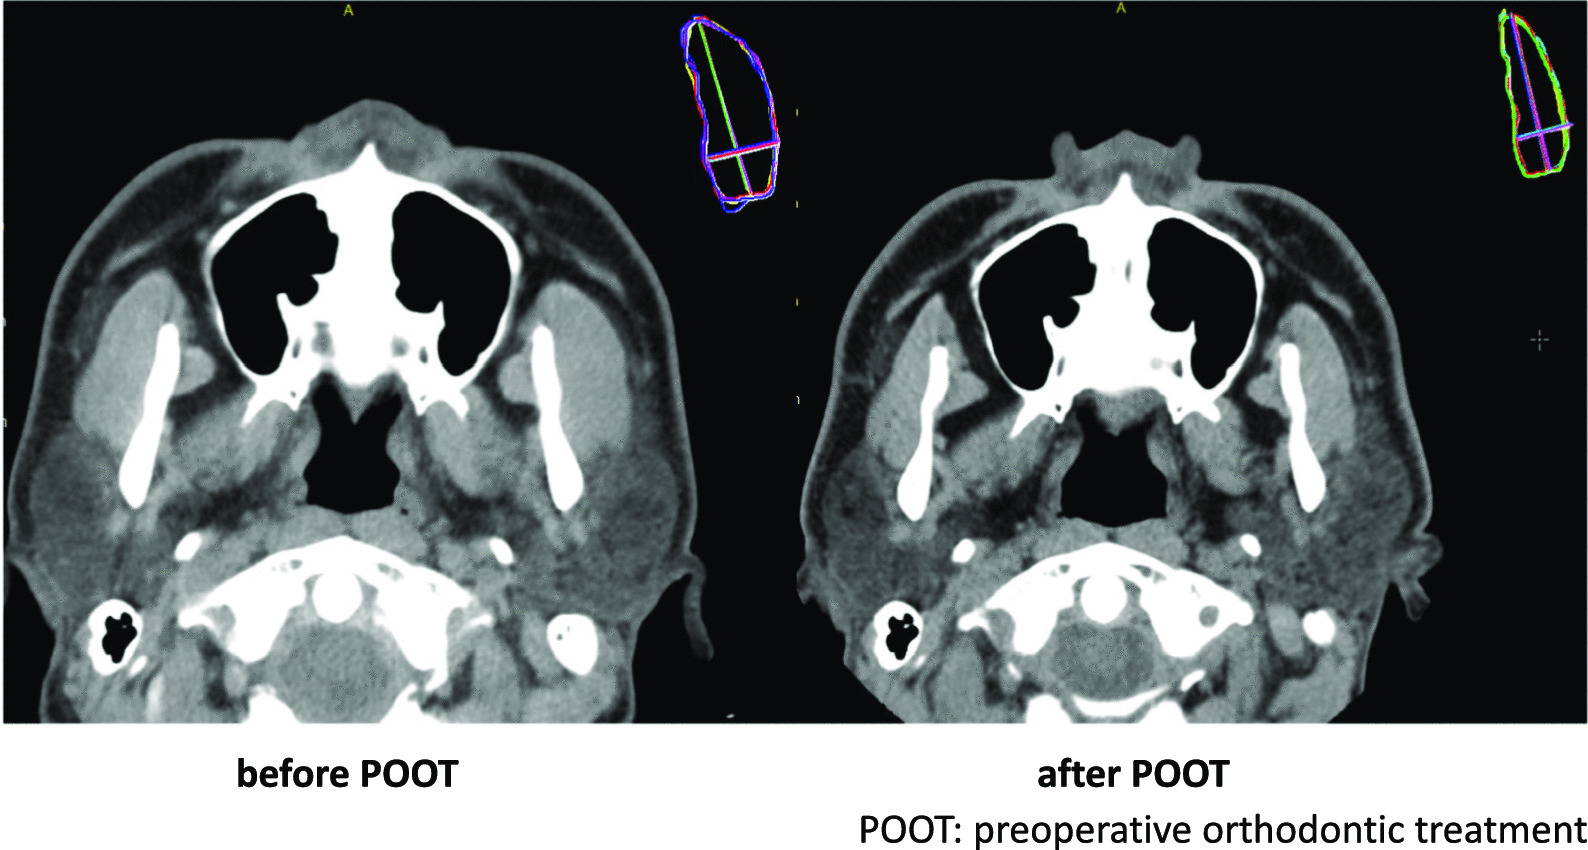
Masseter muscle cross-sec < onal area was measured from 5 mm above the mandibular foramen parallel to the Frankfurt plane using CT images
